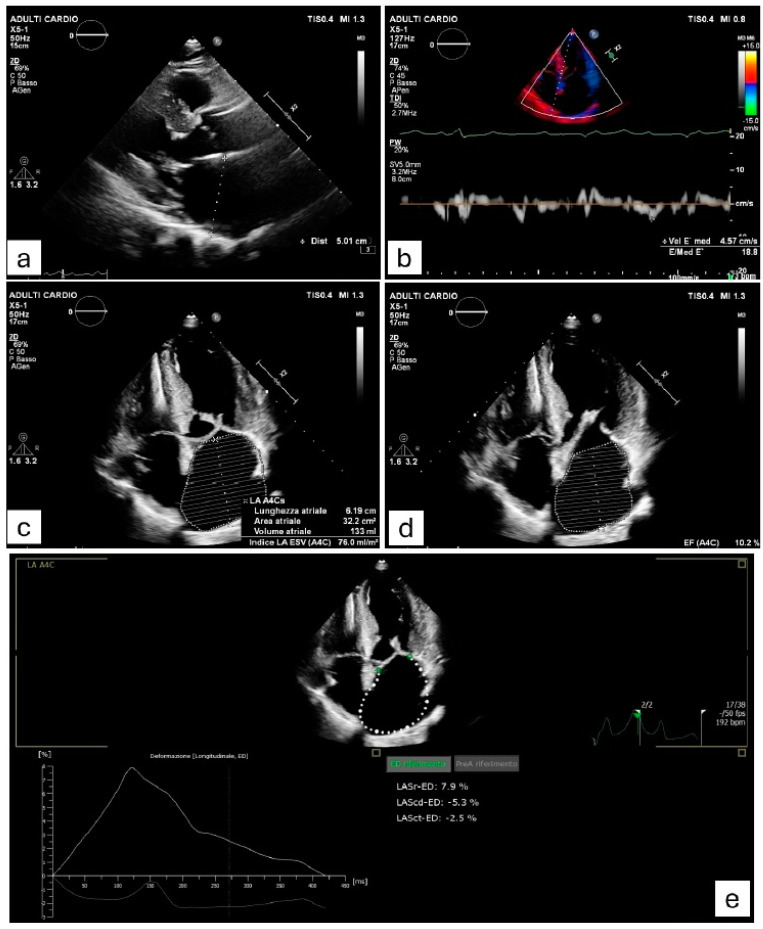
Clinical case of a patient affected by hypertrophic cardiomyopathy (HCM) who experienced a paroxysmal episode of atrial fibrillation (AF). The image shows echocardiographic measurements of various variables which have been demonstrated to be associated with AF onset in HCM. (a) Severely increased left atrial antero-posterior diameter (≥45 mm). (b) E/e’ ratio ≥ 17, suggesting elevated left ventricular filling pressures. (c) Marked dilation of left atrial area (≥28 cm^2^) and left atrial volume index (≥34 mL/m^2^). (d) Impaired left atrial ejection fraction (≤45%). (e) Alterations in the three left atrial phasic functions (reservoir, conduit, pump), assessed by strain-derived measures.
